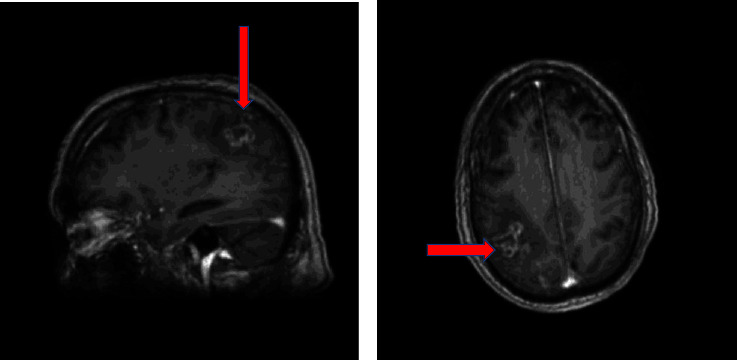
(a, b) MRI of the brain with contrast medium shows a non–space-occupying lesion in the right parietal region with perifocal edema in the sagittal and axial views in the cMRI images without typical signs of CM (red arrow).
